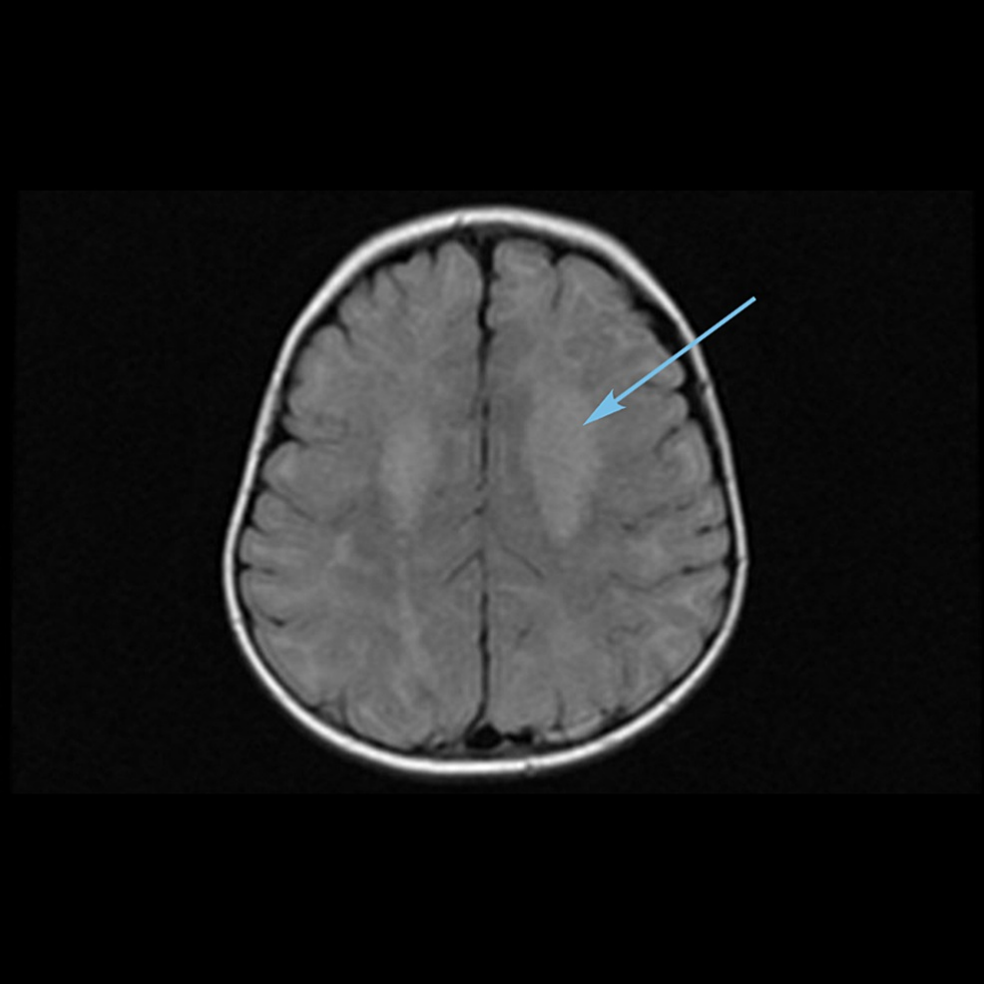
MRI brain axial T2-weighted image showing hyperintensities in centrum semiovale (arrow).
